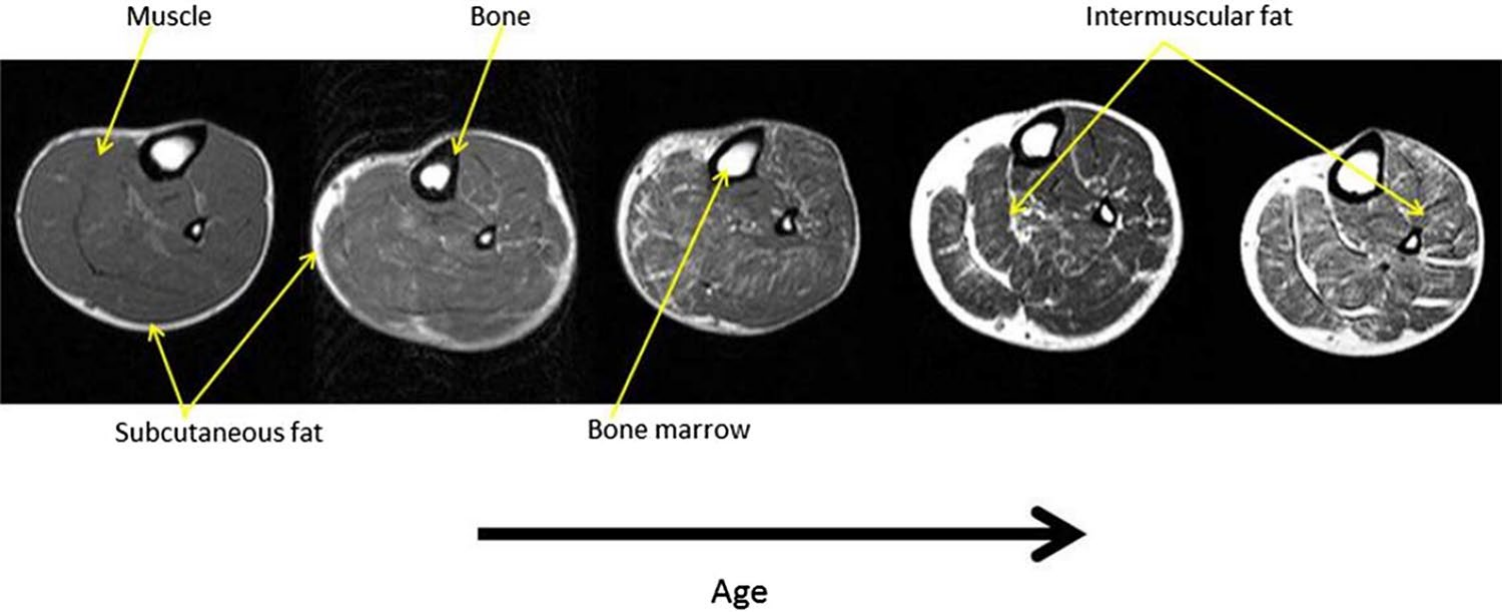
Axial MR images from the lower leg of 5 volunteers, showing typical changes arising as a result of the normal aging process. This includes both a reduction in the proportion of ‘muscle mass’ and the ‘quality’ of the muscle, with a visible increase in the amount of intermuscular fat deposited
